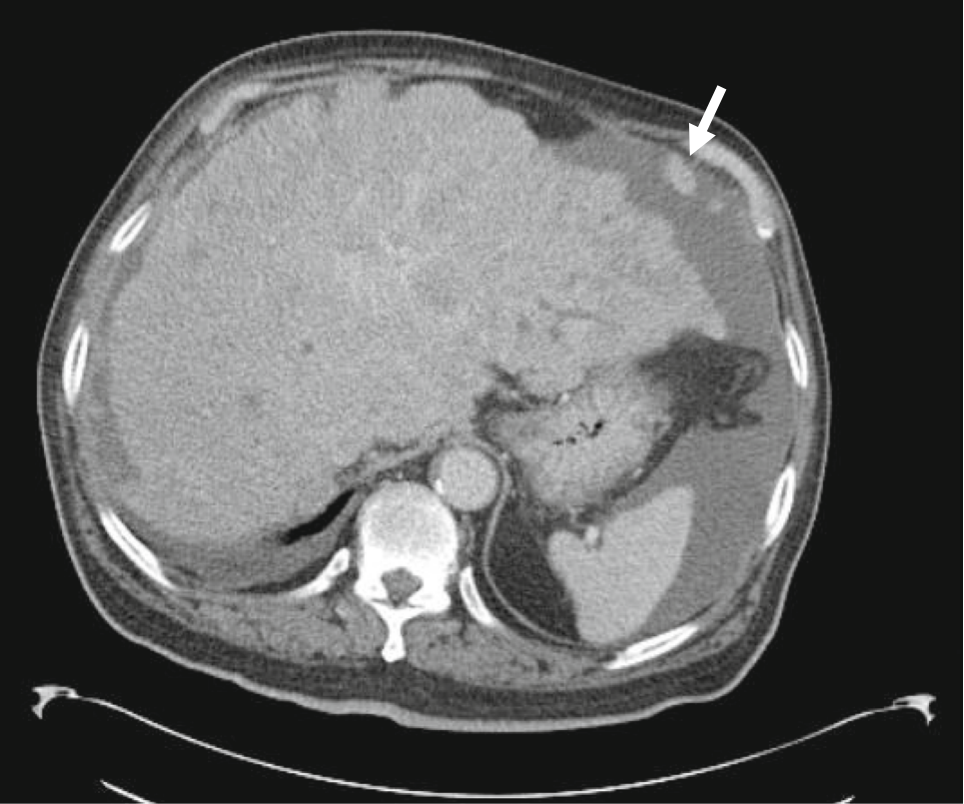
Portal venous phase CT demonstrates an enlarged liver with a markedly irregular contour due to metastatic melanoma. The patient has ascites and peritoneal deposits (*arrow*), and clinically was heavily jaundiced, with gross hepatic dysfunction. Note normal-sized spleen
